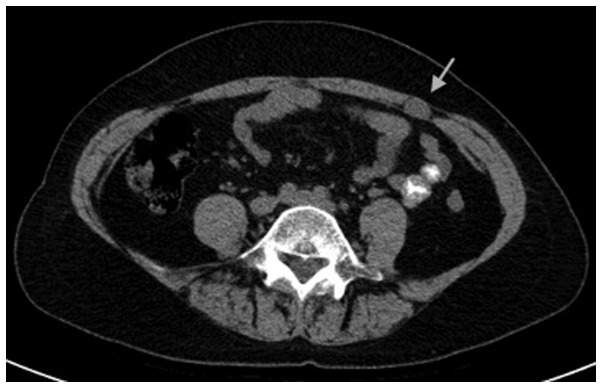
Basal computed tomography examination revealing a small, oval-shaped, omogeneous mass, with a soft tissue density that can be observed on the abdominal wall, on the left edge of the left rectus abdominis muscle. The mass possesses regular edges and is well-demarcated by fat from the sourrounding structures (white arrow).
